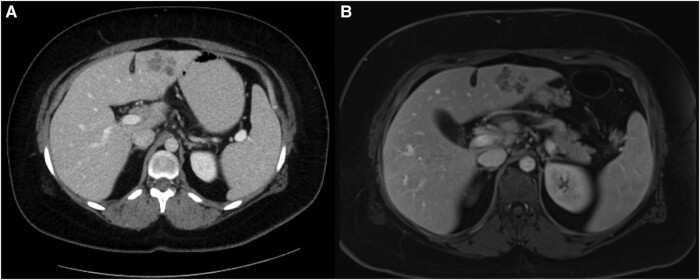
A*, Hepatic abscesses on abdominal axial view of computed tomography show multiple small abscesses in segment 2/3. *B*, Hepatic abscesses on T1-weighted magnetic resonance imaging axial view measure 2.9 × 3.6 cm in segment 3.
